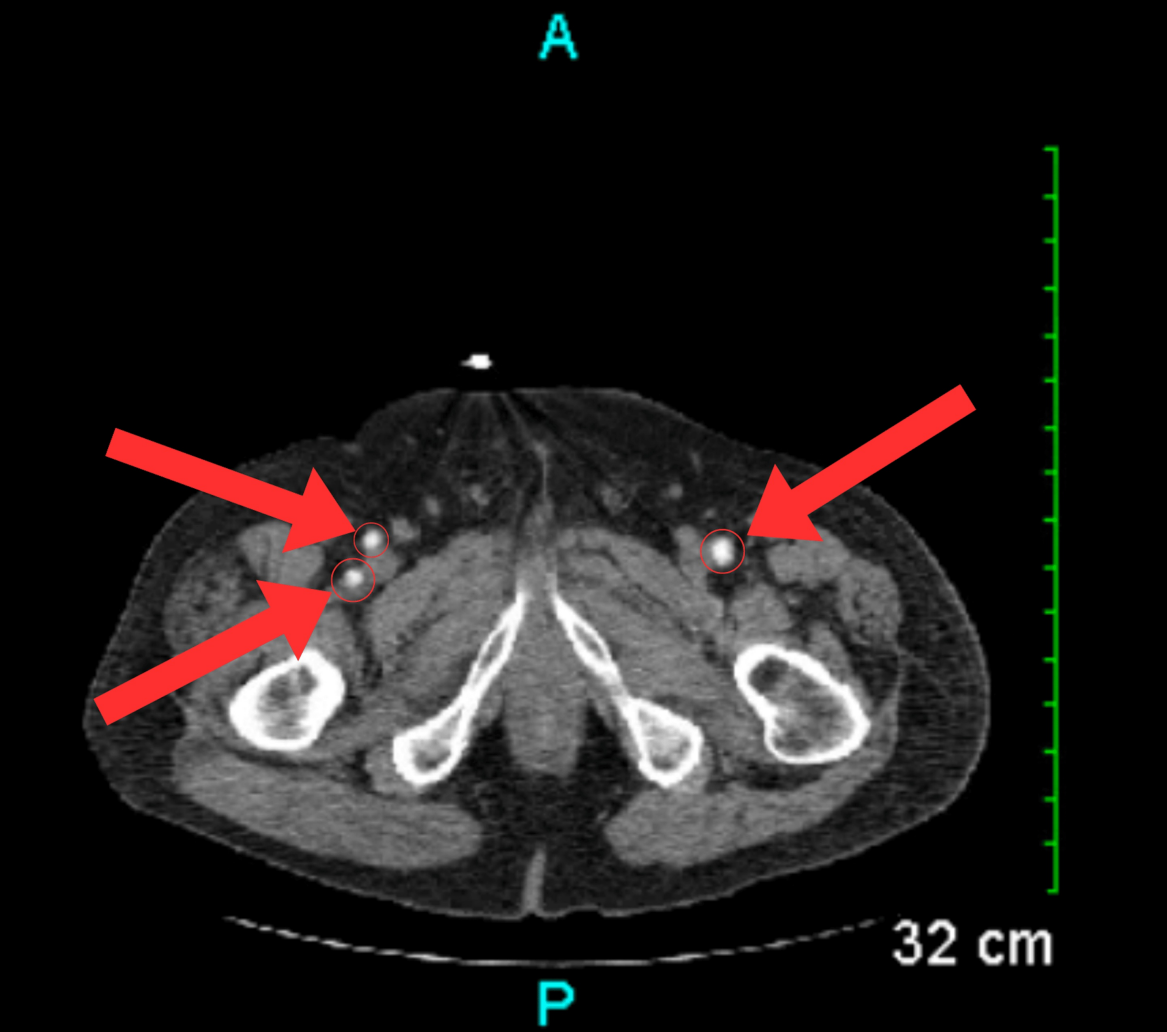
CT scan showing pelvic phleboliths (red arrows and circles). CT: Computed tomography
